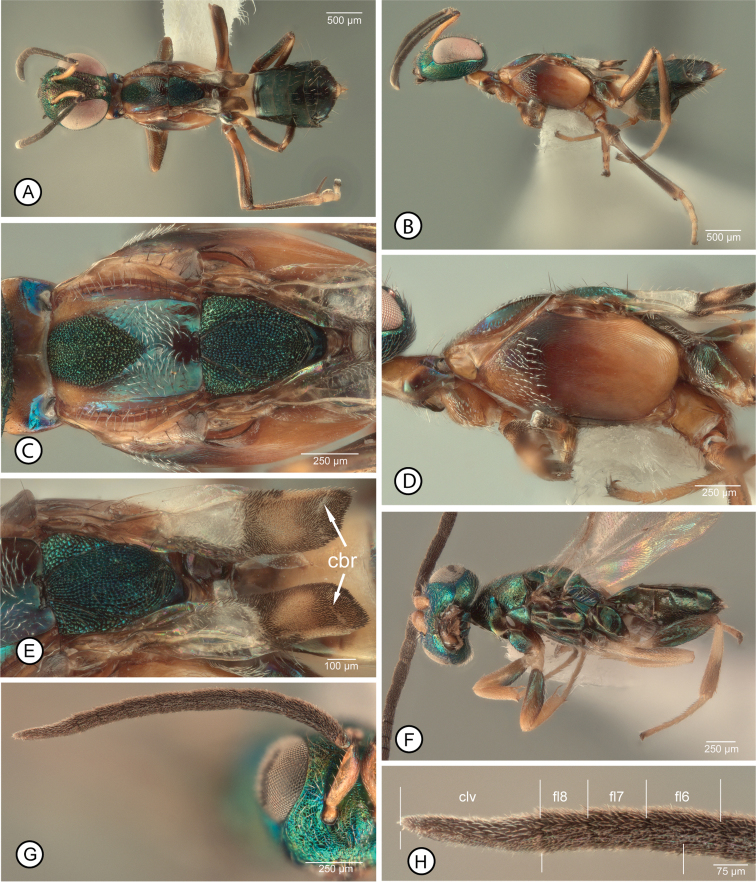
Anastatusmeilingensis*A–E female (2): A dorsal habitus B lateral habitus C dorsal mesosoma D lateral mesosoma E fore wing, dorsal view. F–H male (19): F lateral habitus G antenna H clava and apical three funiculars (three lower bars indicate length of clava compared to combined length of apical funiculars). Abbreviations: cbr = remnant of hyaline cross band, clv = clava, flx = flagellomere number.
